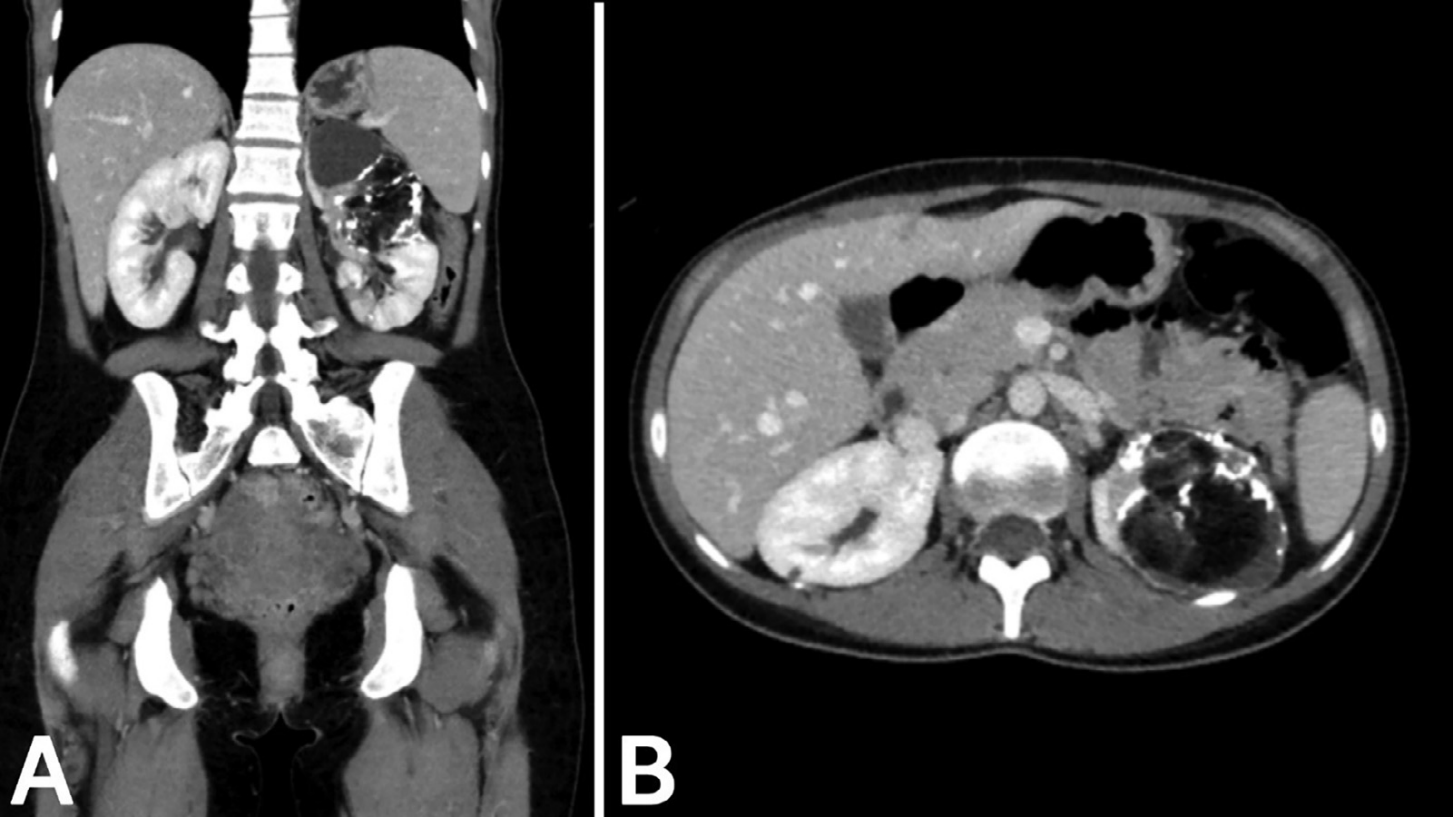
Post-TAE CT with contrast findings. A: Coronal plane in the portal phase showing the resolved hematoma and lipiodol distribution within the AML in the left kidney, confirming targeted embolization. B: Axial plane in the portal phase showing the decreased size of the AML and absence of residual enhancement, indicating successful TAE.
